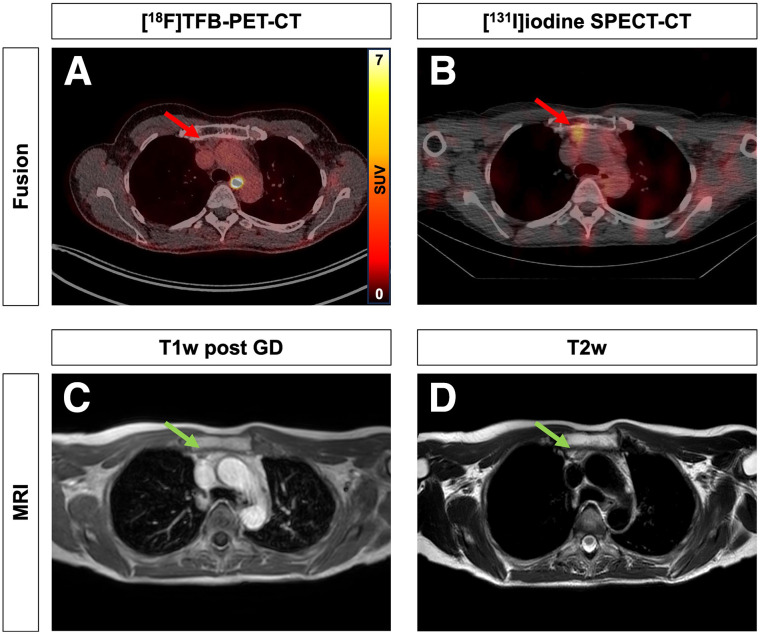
False-positive thymus uptake in [^131^I]iodine SPECT/CT. 49-y-old woman with follicular thyroid cancer. Absent [^18^F]TFB uptake (A) in [^131^I]iodine-positive (B) mediastinal mass (red arrow). Subsequent contrast-enhanced MRI showed thymus rebound (green arrow) in T1-weighted (after gadolinium) (T1w post GD) and T2-weighted (T2w) (C and D) sequences. [^131^I]iodine uptake therefore was considered to be false-positive, a conclusion that was also confirmed by further follow-up.
